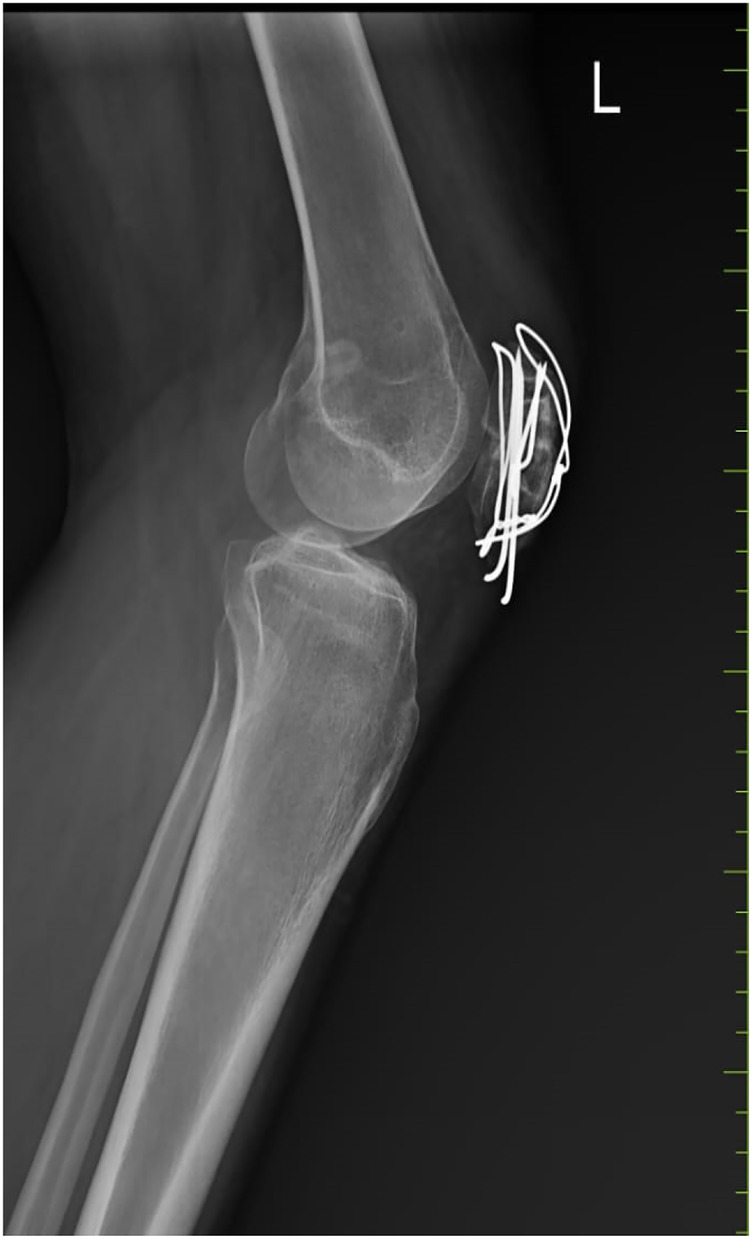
Shows the radiographs reveal a previously displaced transverse fracture of the patella that has been surgically stabilized.
